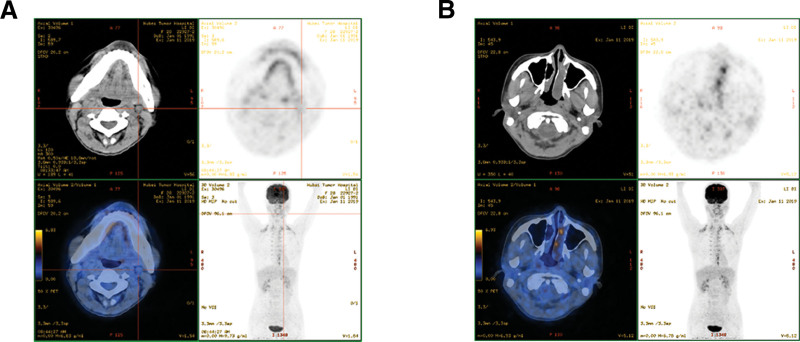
Images of whole-body positron emission tomography-computed tomography (PET-CT) performed after partial lymphadenectomy. (A) In contrast to images of the first PET-CT, the images of the second PET-CT showed that the residual submandibular lymph nodes had reduced in size (the maximum diameter was about 0.8 cm). (B) A new concealed mass emerged in the left nasal cavity.
